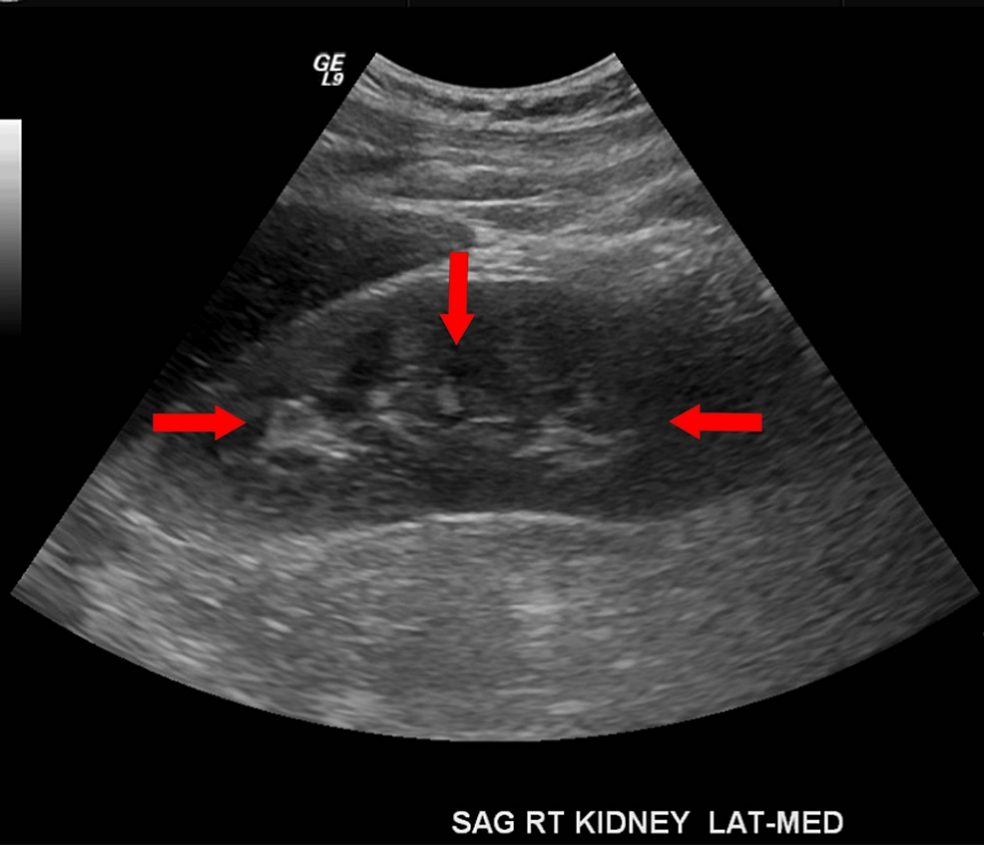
Renal ultrasound showing an echogenic kidney consistent with chronic medical renal disease (as indicated by arrows).
